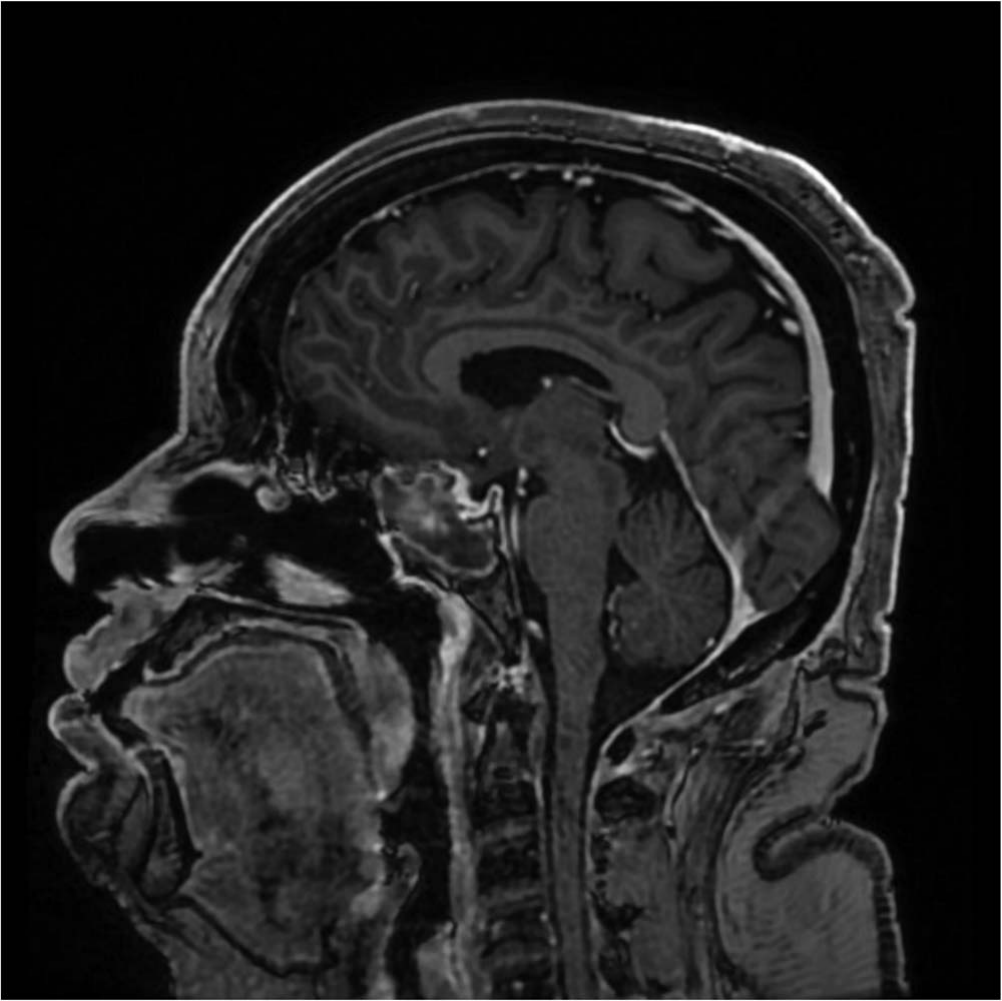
A sagittal brain MRI reveals the same mass with superior extension to the sellar and suprasellar regions. The lesion is exerting a compression to the optic chiasm. The mass effect extends posteriorly toward the clivus, with irregular margins indicating invasive potential. Adjacent structures like the pituitary stalk appear to be displaced.
